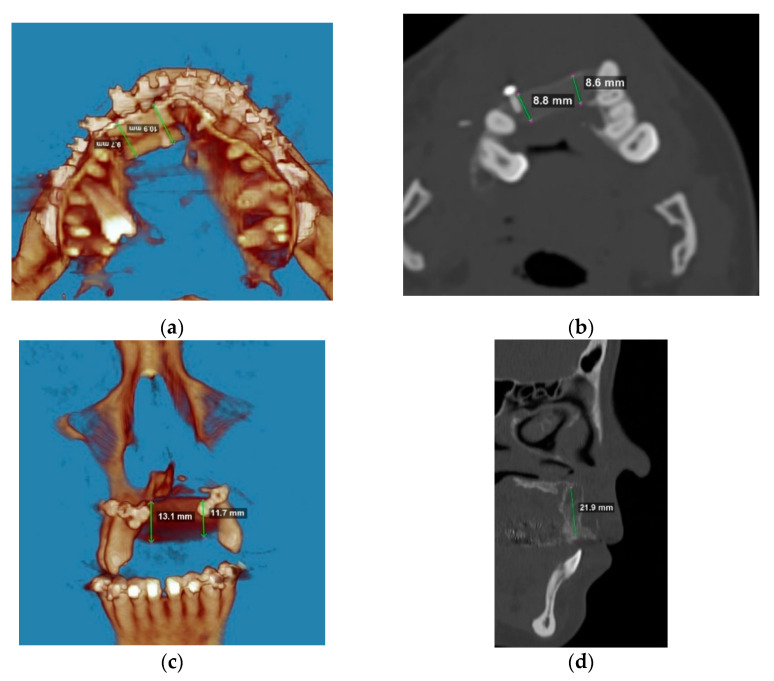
Representative photographs and 3D CT scans used for measurements of the graft: (a) 3D reconstruction in horizontal plane, (b) horizontal plane CT scan, (c) 3D reconstruction in coronal plane, (d)lateral plane CT scan.
